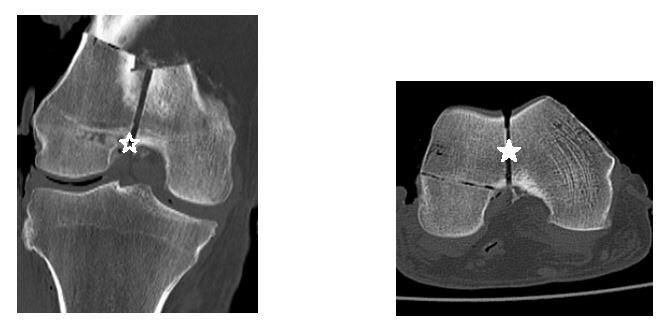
An example PowerPoint slide of two CT scan slices used for measuring fracture displacement. Each reviewer was asked to measure the amount of displacement in both the coronal (open star) and axial (solid star) planes at the level of the articular surface. An average of these two measurements was then recorded.
